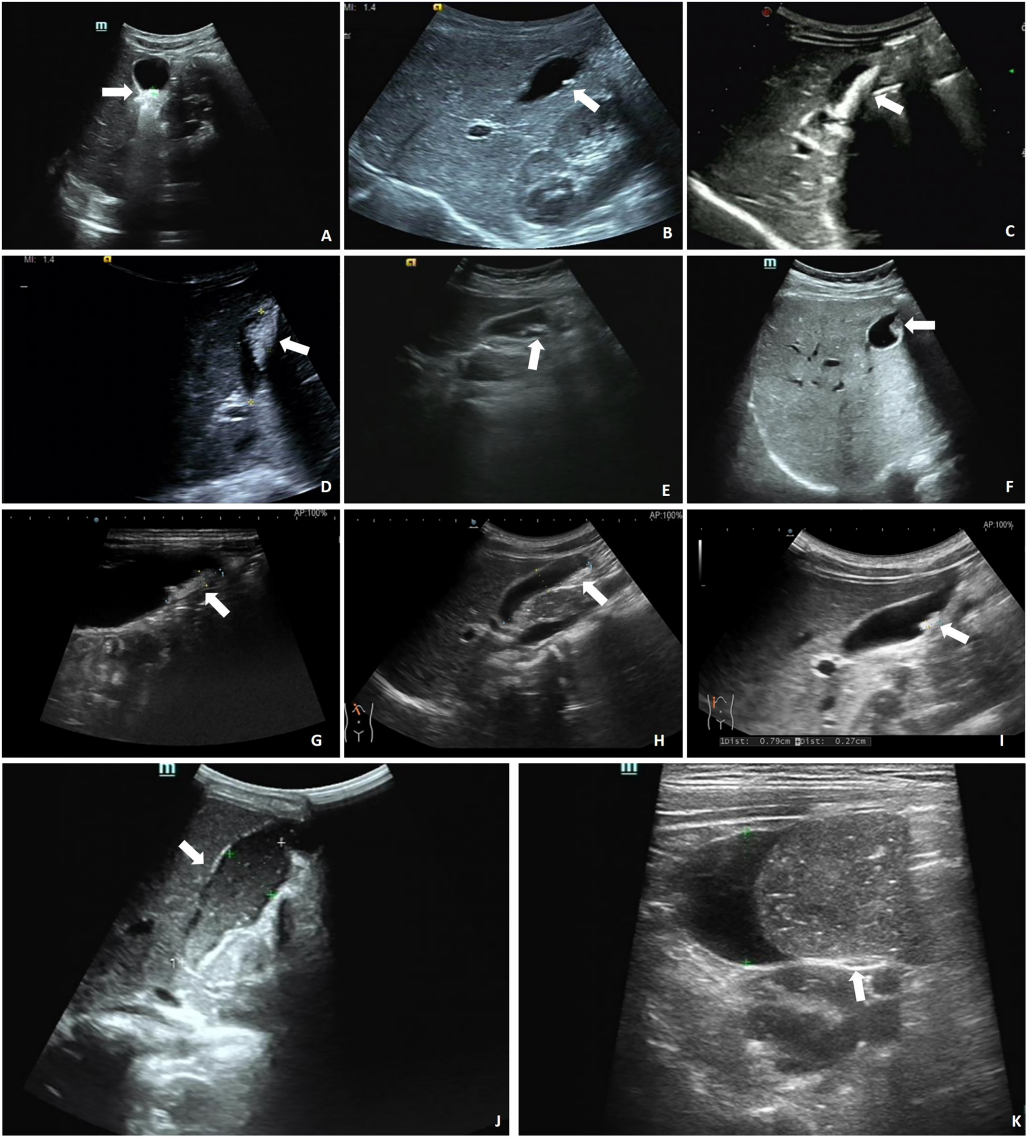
Ultrasound in the gallbladder. (A) 0.8 × 0.4 cm strong echo. (B) 0.6 × 0.2 cm strong echo. (C) Sand-like echoes, 2.6 × 0.7 cm. (D) Sand-like echoes, 2.2 × 1.1 cm. (E) Sand-like echoes, 2.2 × 0.4 cm. (F) Accumulated strong echoes, 1.2 × 0.7 cm and 1.0 × 0.5 cm. (G) 1.6 × 0.4 cm strong echo. (H) 1.9 × 0.5 cm strong echo. (I) 0.8 × 0.3 cm strong echo. (J) 5.6 × 2.1 cm low echo. (K) 5.6 × 1.9 cm low echo. Arrows indicate strong/low echoes in the gallbladder.
